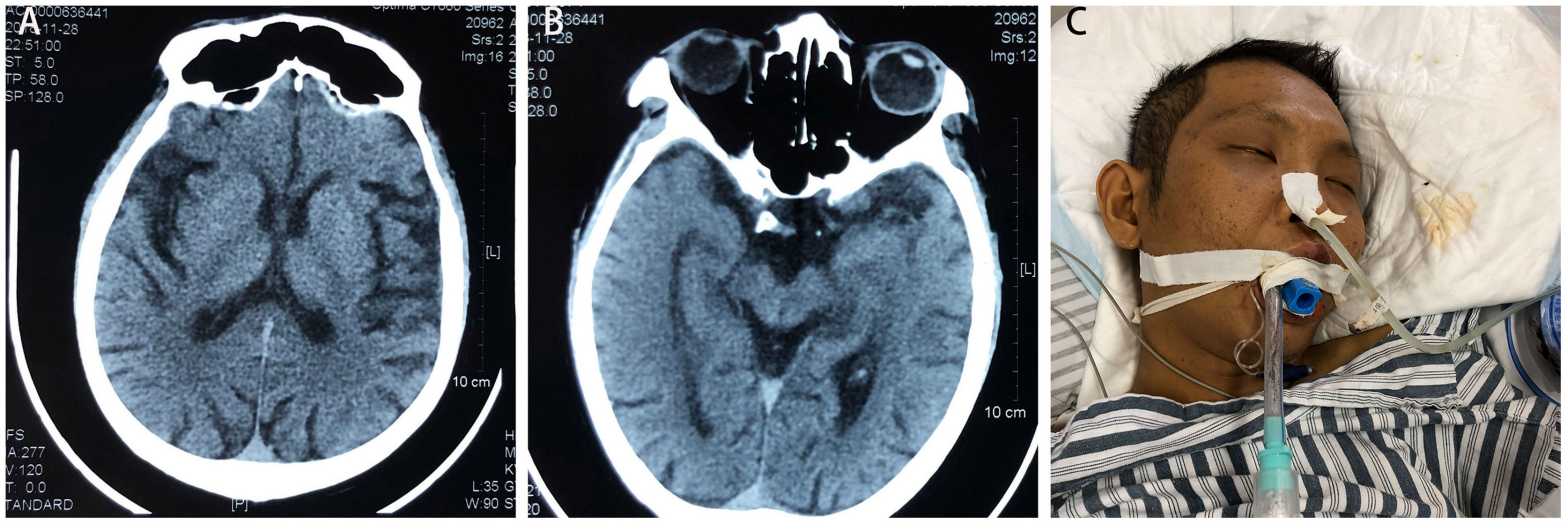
Craniocerebral computed tomography scan indicates wide and deep cerebral sulcus, flattened cerebral palsy, expanded ventricles, thickened cerebellum and reduced brain volume (A,B). (C) The patient was placed on a ventilator but soon died of persistent epilepsy and multiple organ failure.
